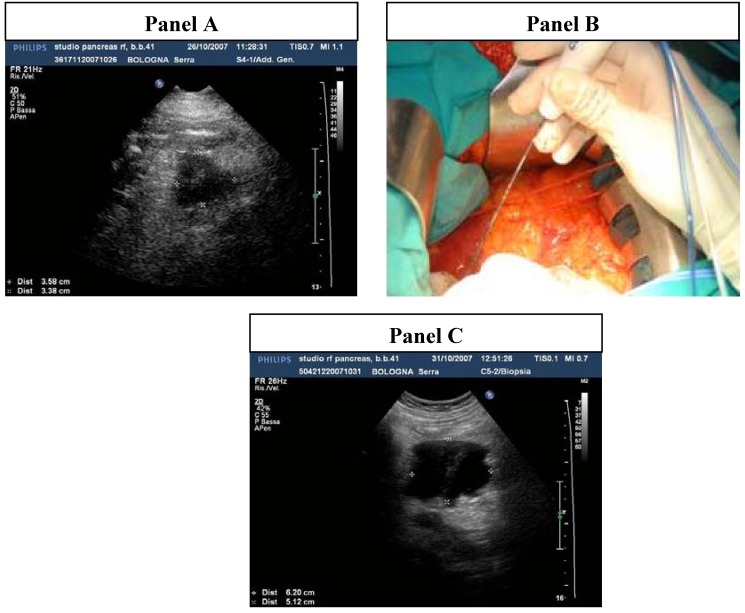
Radiofrequency ablation (RFA) and ultrasonographic (US) evaluation of the technique in a patient with locally advanced pancreatic head carcinoma. Panel A*.* Intraoperative US showing the pancreatic adenocarcinoma; Panel B*.* Intraoperative RFA; Panel C. Intraoperative US showing the ablated carcinoma.
